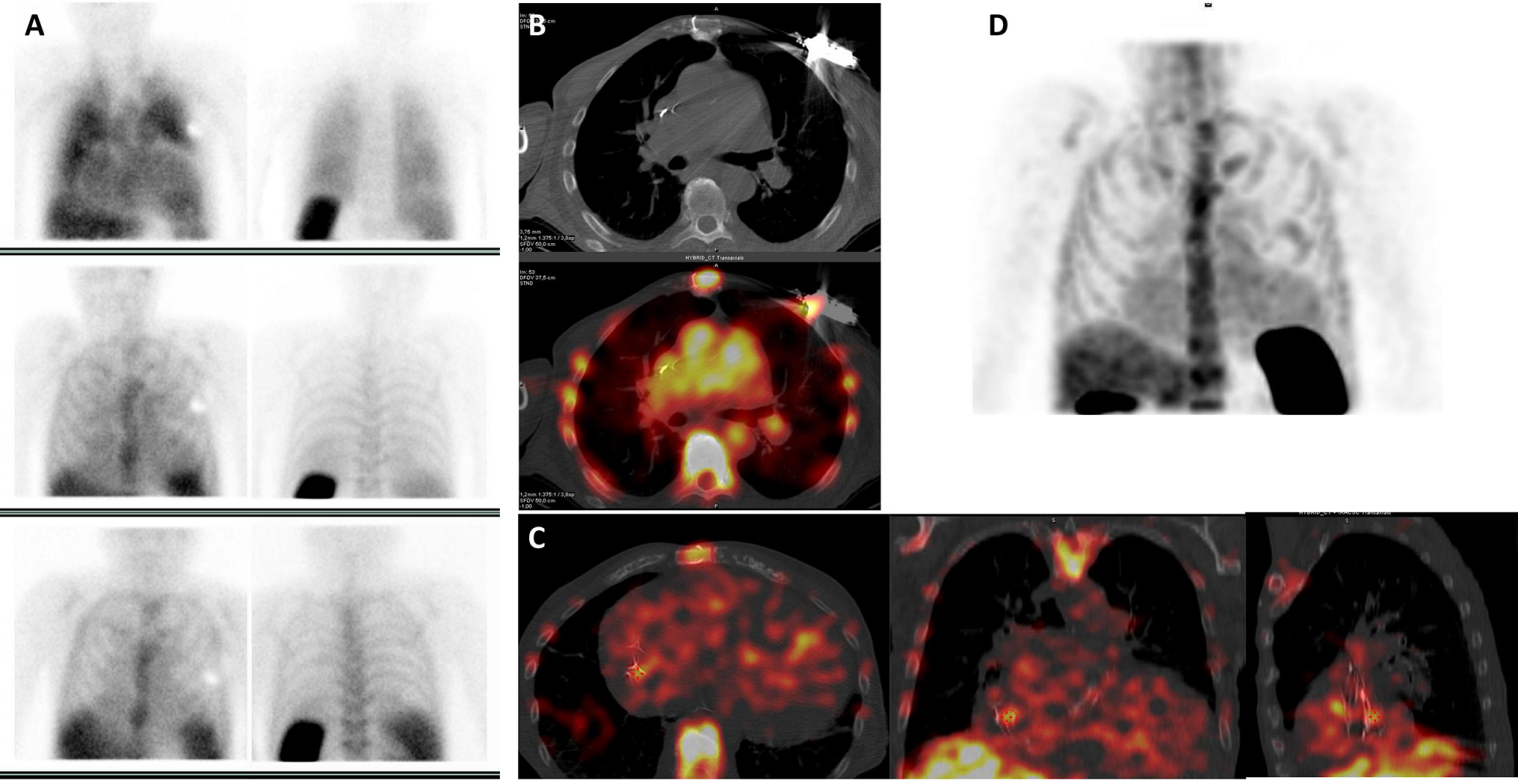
Radiolabeled leukocyte scintigraphy (^99m^Tc-HMPAO WBC) in a patient with suspected CIED infection. Planar images (A, top-down at 30 min, 6 h, and 20 h) after the injection of the radiopharmaceutical show an area of pathological leukocyte accumulation that at SPECT/CT (B, transaxial CT and superimposed SPECT/CT and D, MIP images) is localized at the level of the ICD pocket in the deep posterior portion. In addition, an area of radiopharmaceutical uptake is also evident at the level of the electro-catheter in the right intravascular and interatrial tract (C, superimposed SPECT/CT in transaxial, coronal, and sagittal views, respectively)
